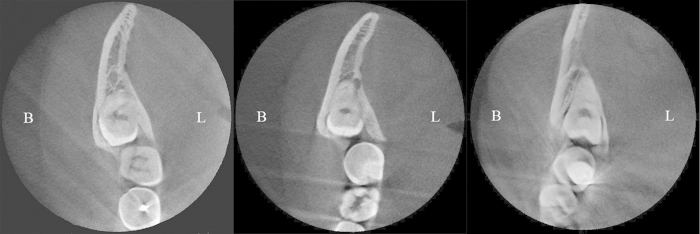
Classification of mandibular third molar’s position based on buccal-lingual alveolar bone thickness, as seen on CBCT parallel sections. (A) Buccal position. (B) Central position. (C) Lingual position.
